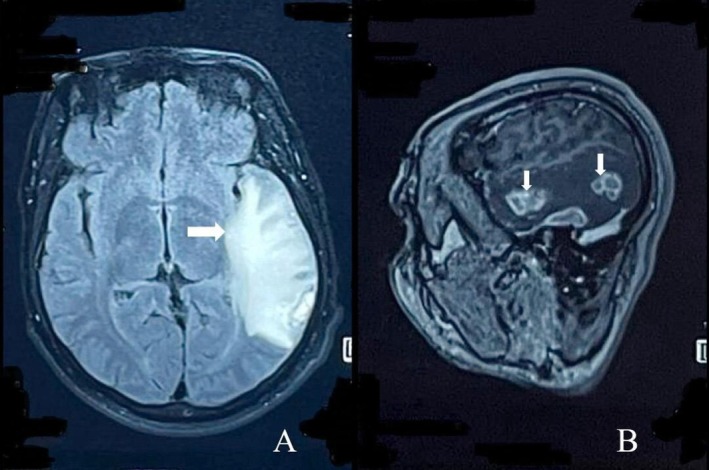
Brain MRI of a 40‐year‐old virologically suppressed HIV patient with Primary CNS lymphoma. (A) Axial T2/FLAIR image showing a large, ill‐defined hyperintense lesion in the left temporal lobe with vasogenic edema and mild compression of the left lateral ventricle. Subtle rightward midline shift is noted. (B) Sagittal post‐contrast T1‐weighted image demonstrating multifocal irregular ring‐enhancing lesions corresponding to the temporal lobe mass with marked vasogenic edema, highly suggestive of primary CNS lymphoma.
